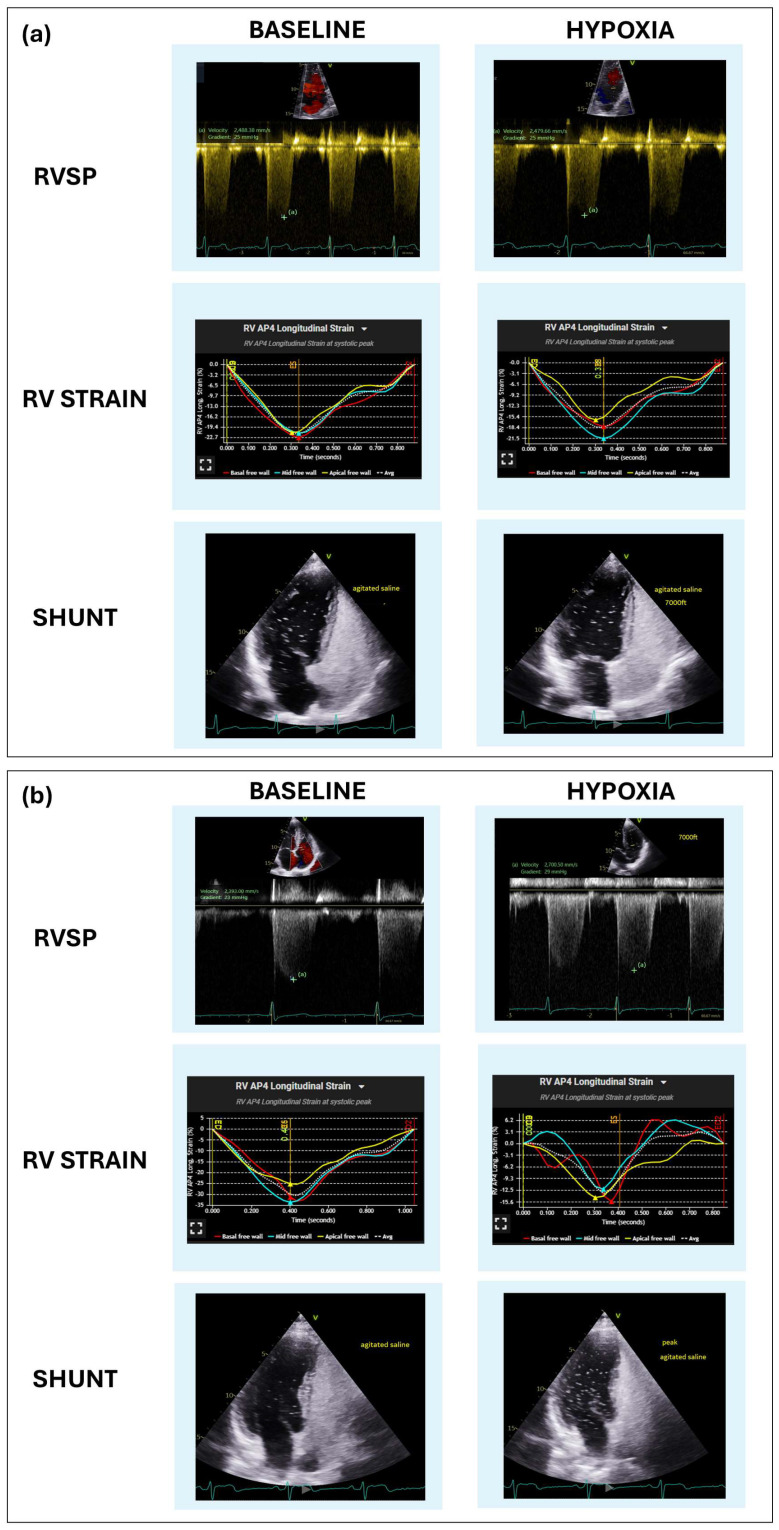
Graphical representation of shunt assessment ("innocent" vs. "guilty") in the hypoxic stress test without exercise and TTE. From top to bottom in panels (a) and (b), apical 4−chamber view TTE images during the contrast bubble test, results of RV longitudinal strain and tricuspid regurgitation jet for calculating RVSP before and during the peak of hypoxic stress. (a) Small shunt, without increased its magnitude and minimal modifications in hemodynamic parameters and oxygen saturation under hypoxia (“innocent” shunt). (b) Small shunt that with an increased under hypoxia and significant oxygen desaturation, increase in RVSP and a fall in RV longitudinal strain and (“guilty” shunt).
